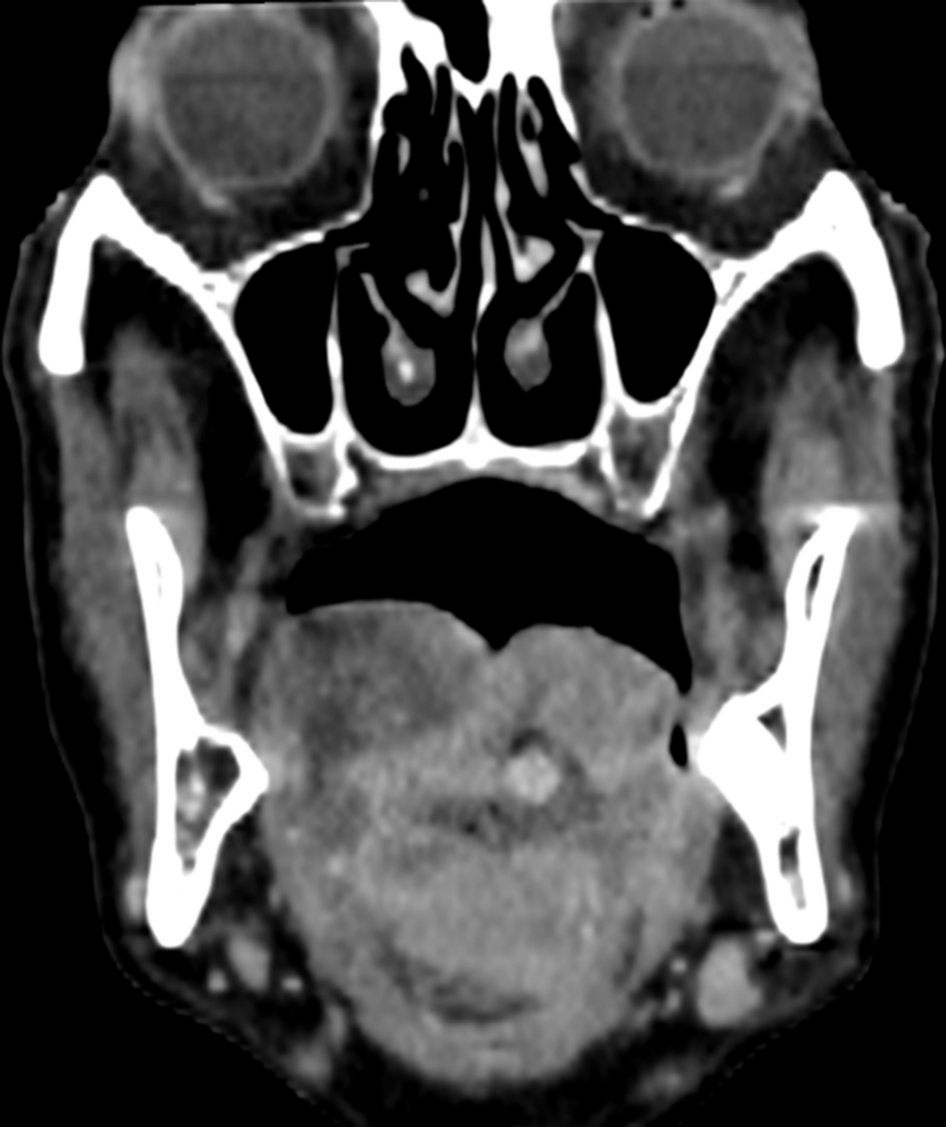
Coronal venous CT image, revealing the extension of the lesion to the two posterior thirds of the tongue and an enhancing pseudoaneurysm within the necrotic/ulcerative centre of the mass.
